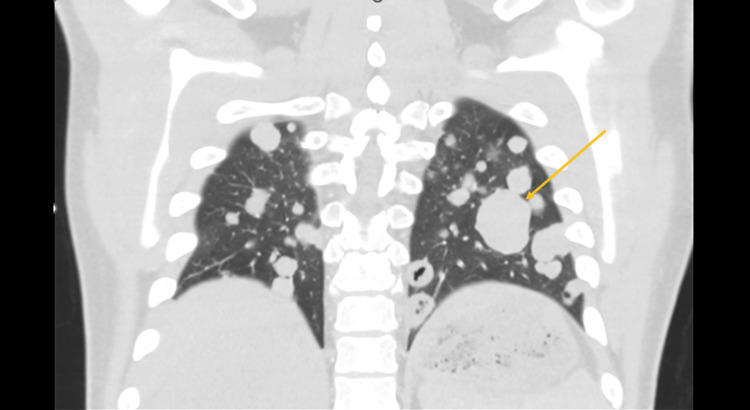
CT scan of the chest revealed bilateral pulmonary nodules, the largest measuring 1.9 cm in the right middle lobe, which suggested hematogenous dissemination.
